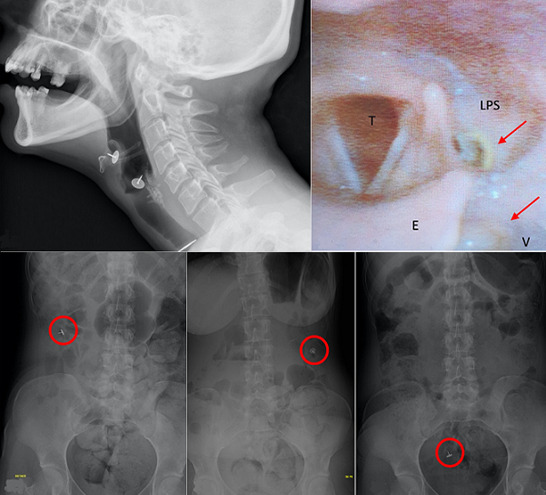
A) lateral neck x-ray; two foreign bodies are identified (thumbtacks); B) fibroscopic evaluation; C) progression of the thumbtack not removed endoscopically (day 2, 5 and 7 respectively) (E: epiglotis; LPS: left pyriform sinus; T: trachea; V: valeculae)
